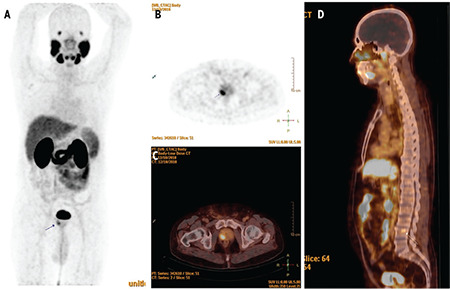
The prostate-specific membrane antigen positron emission (Ga-68 PSMA PET) image of 67 years old prostate carcinoma patient (A), transraxial PET/computed tomography (CT) image of Ga-68 PSMA expressing primary tumor in right lobe apex (B), transraxial PET/CT fusion image (C), sagittal plan PET/CT image of metastatic lesion in the lumbar 4^th^ spine expressing Ga-68 PSMA (D) PSMA: Prostate-specific membrane antigen, PET: Positron emission tomography, CT: Computed tomography
